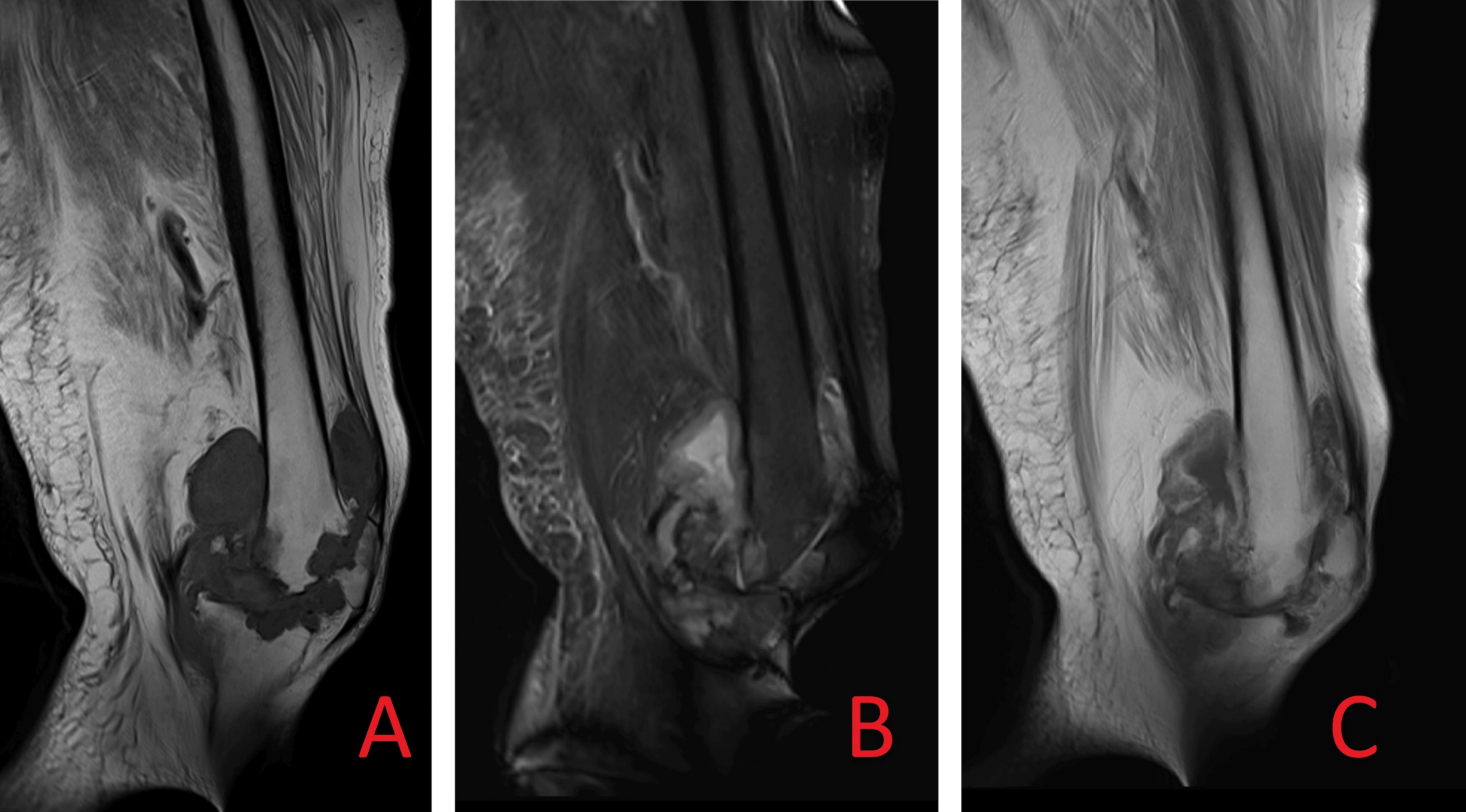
The MRI scans demonstrate irregular areas of soft tissue signal intensity, which are T1 hypointense and PDFS hyperintense, showing post-contrast enhancement seen extending into joint space. 3A: T1-weighted MRI; 3B: T2-weighted MRI; 3C: post-contrast MRI PDFS: proton density fat-suppressed
